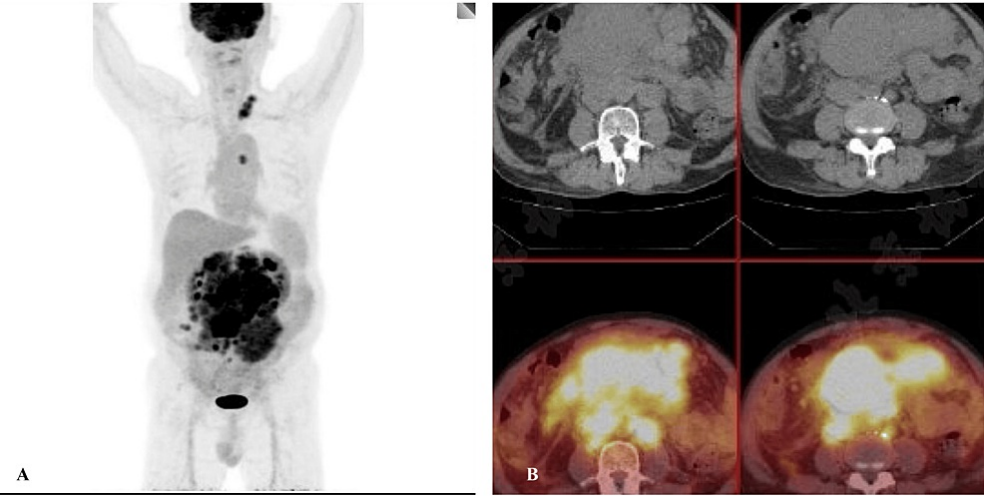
PET/CT PET/CT imaging displays numerous abnormal radioactive uptake masses and nodules located in the retroperitoneum, mesenteric area, bilateral common iliac vessels, and presacral area. PET/CT - positron emission tomography-computed tomography
